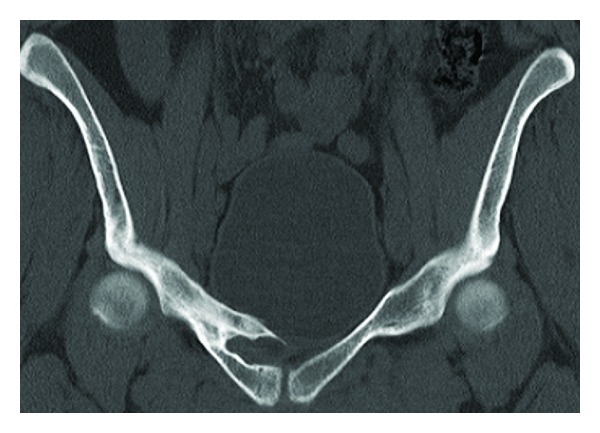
CT coronal slice of the pelvis demonstrating a malunited right superior pubic fracture with a bony spur projecting through the bladder wall.
